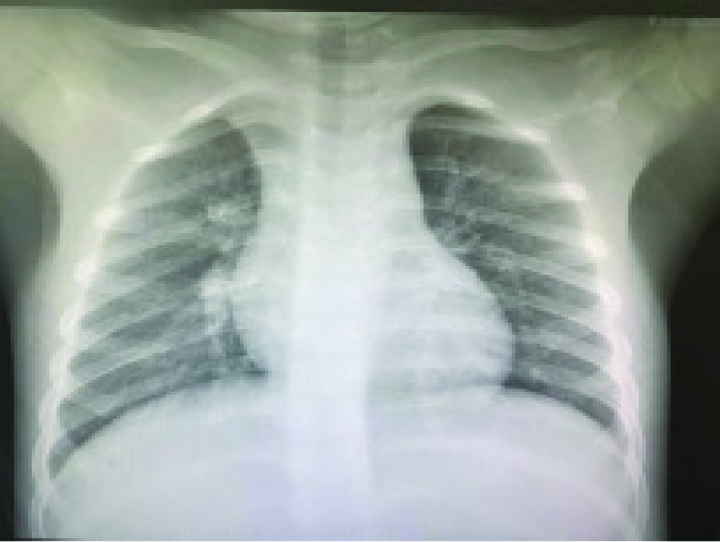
X-ray showing lung marking changes.
